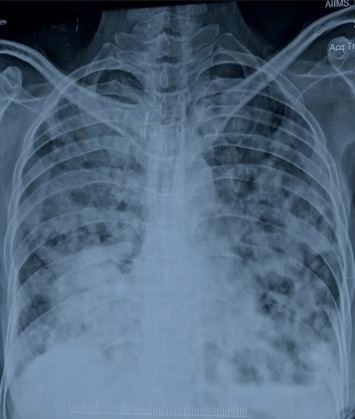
X-ray chest PA view shows bilateral diffuse consolidation and cavitation.
